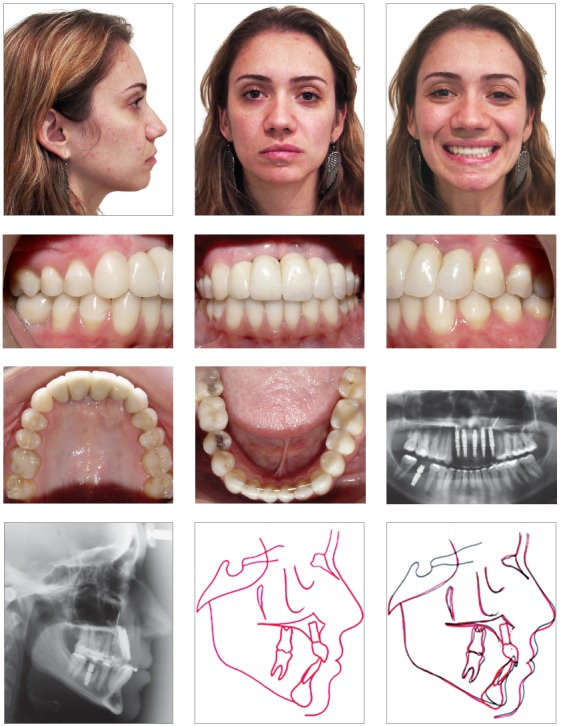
Final photographs, radiographies and superimposition: the prosthesis were placed, and aesthetic and functional rehabilitation was achieved.
